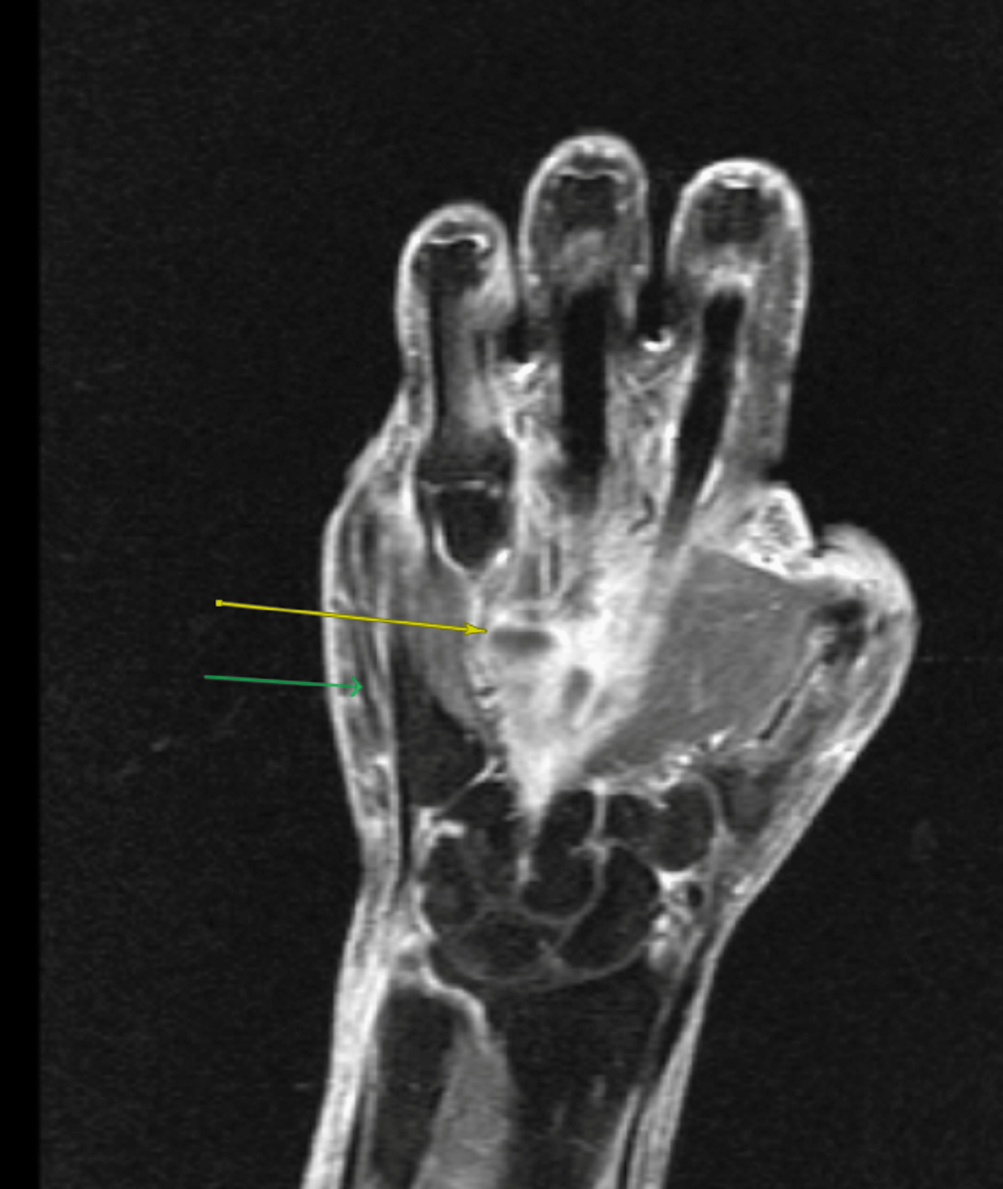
MRI of the right hand showing tenosynovitis from the distal forearm to the palm (yellow arrow), with diffuse subcutaneous edema (green arrow).
